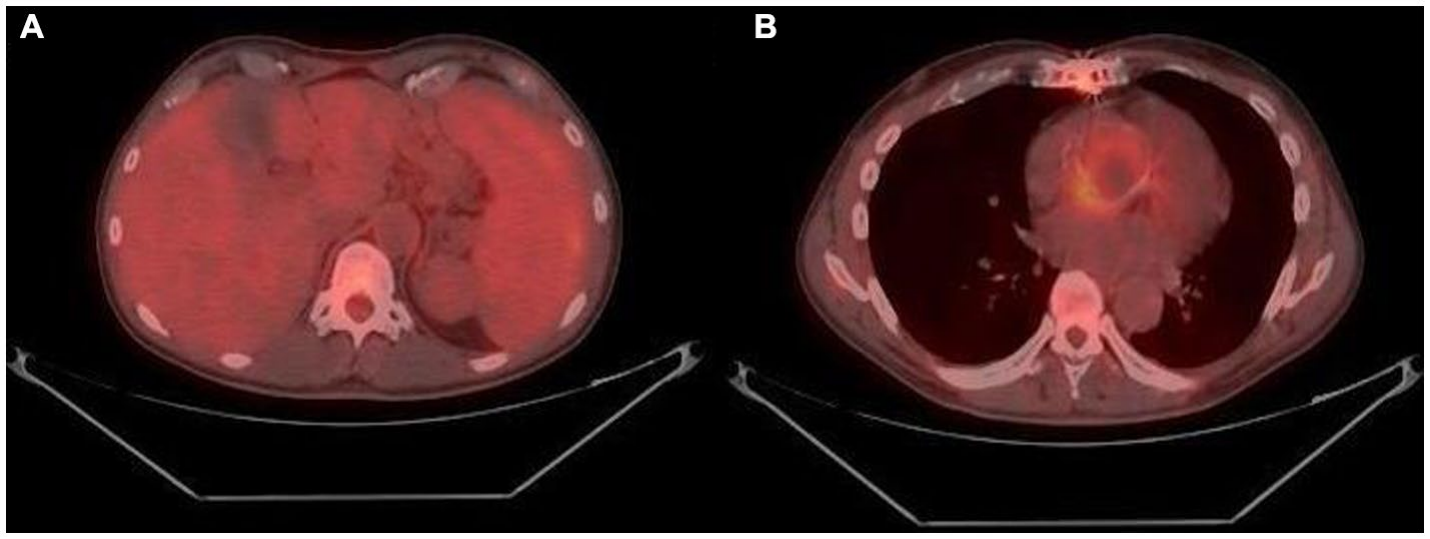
The results of PET-CT: splenic medulla (A) and ascending aorta (B).
